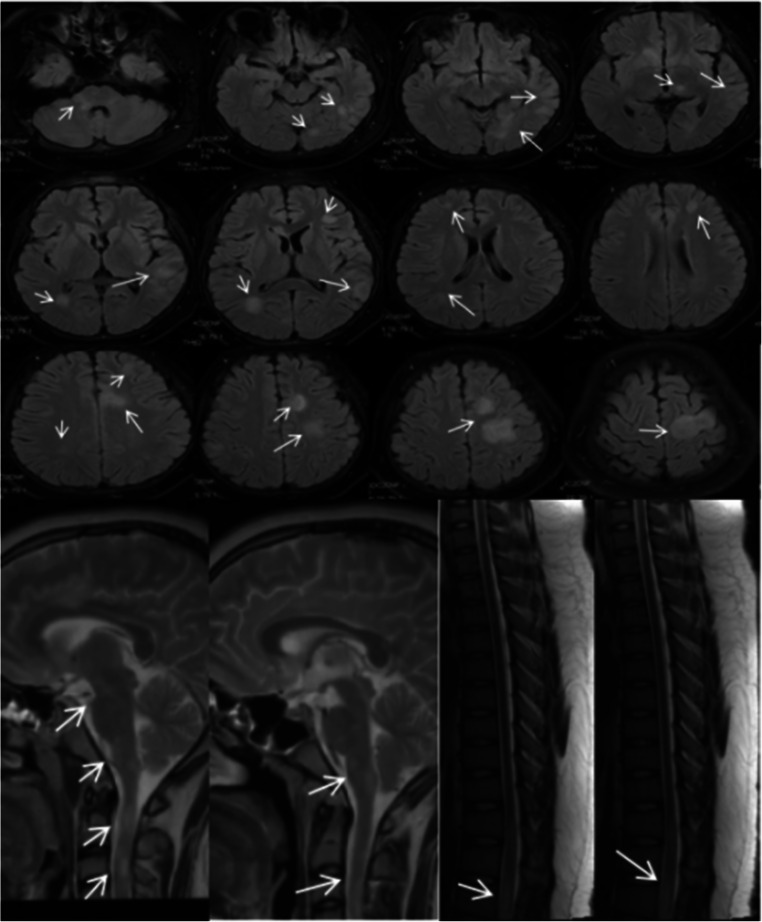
case 1: Brain MRI after symptom recurrence showed multiple intracranial lesions on Flair. Spinal MRI after symptom recurrence showed lesions in the medulla oblongata, spinal, and spinal conus on T2
